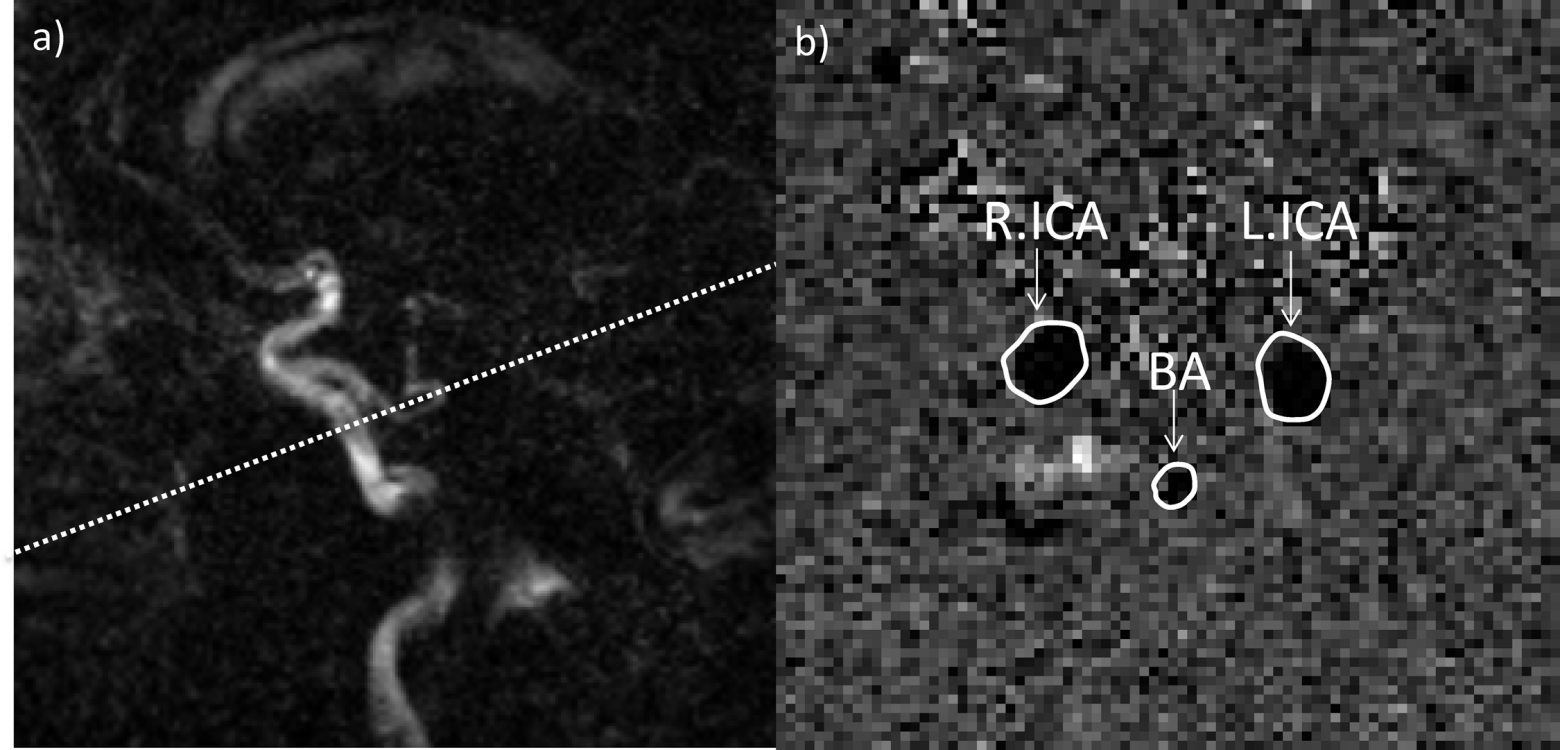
PC-MRI for quantification of total CBF. (a) A PC-MRI scan for measuring mean tCBF was prescribed on a PC-MRI sagittal localizer image perpendicular to the carotid arteries at the level of the mid basilar artery. (b) A representative phase image demonstrating the right and left internal carotid arteries (R.ICA and L.ICA) together with the basilar artery (BA).
